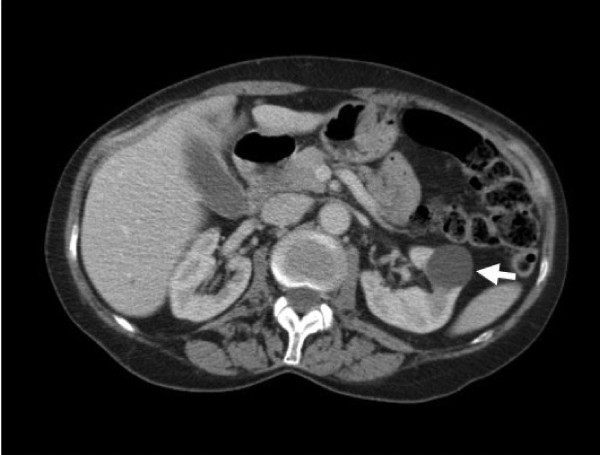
Contrast-enhanced computed tomographic image of the abdomen showing a 1.7 cm non-enhancing lesion in the lower pole of the left kidney (arrow). This was excised by partial nephrectomy and found to be a hybrid oncocytoma, a tumor which is typical of Birt-Hogg-Dubé syndrome.
